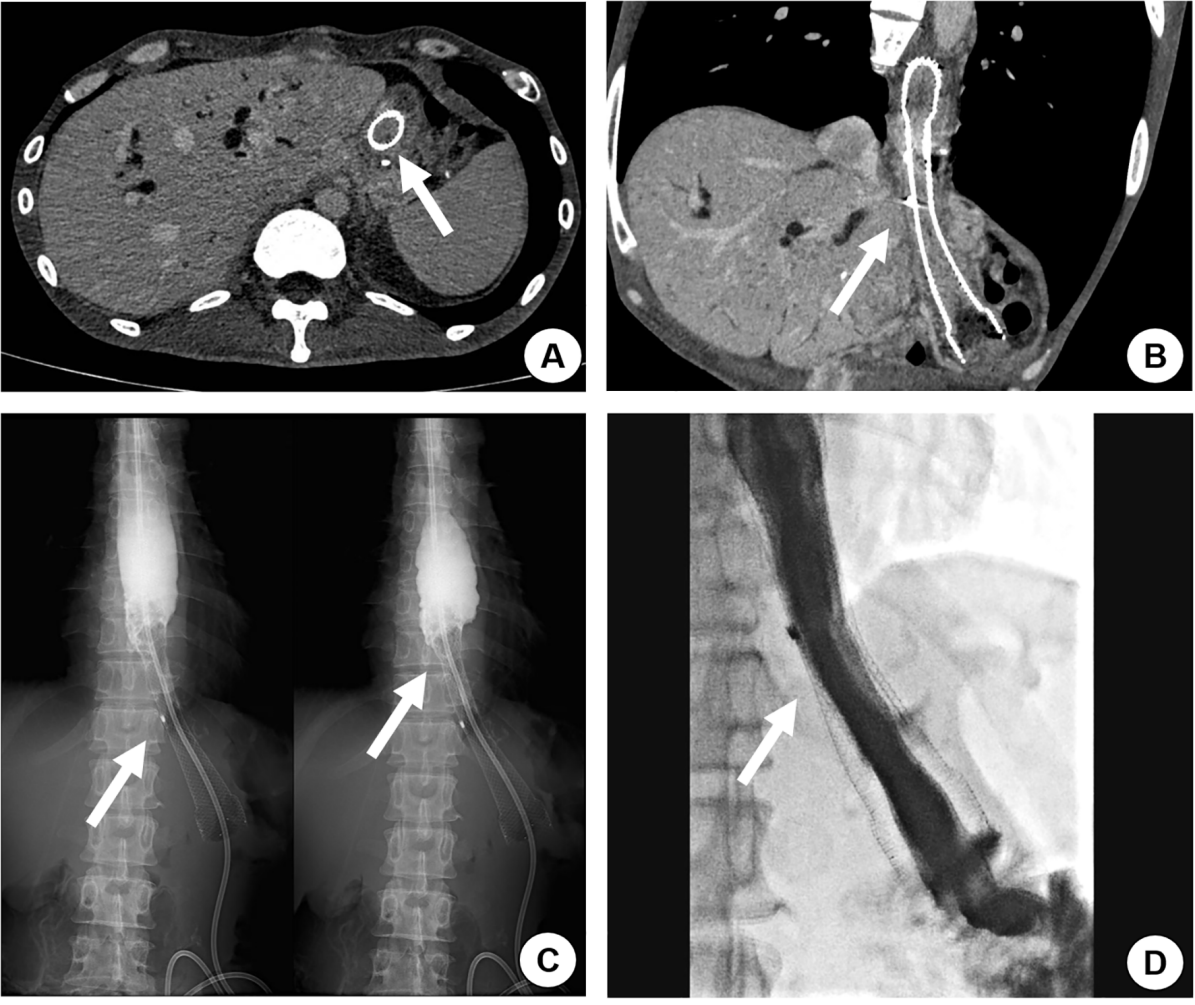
A 42-year-old male with a history of total gastrectomy performed over 1 year prior presented with recurrent nausea and vomiting 131 days after initial self-expanding metal stent (SEMS) placement for esophageal-jejunal anastomotic tumor recurrence with obstruction. Contrast-enhanced CT revealed tumor ingrowth through the stent mesh, causing complete stent occlusion (A, B, arrow). Upper gastrointestinal contrast study confirmed the obstruction, demonstrating no contrast passage beyond the stent (C, arrow). A second overlapping SEMS was deployed, achieving restored luminal patency with free contrast flow into the distal small intestine on repeat imaging (D, arrow). The patient resumed normal oral intake without further obstructive symptoms.
